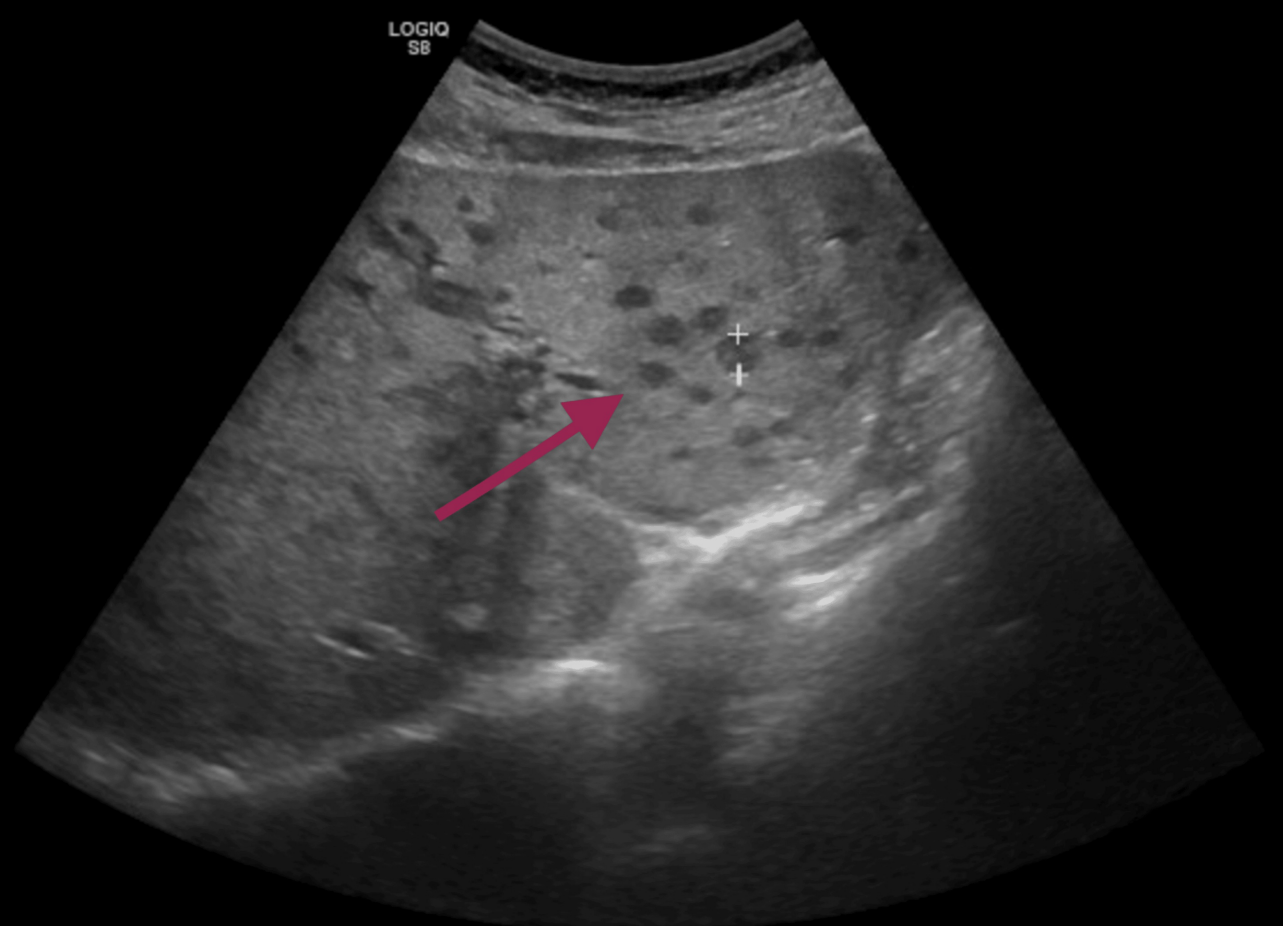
Abdominal ultrasound showing multiple hepatic nodules suggestive of infeccious foci Abdominal ultrasound showing hepatic nodules that were first thought to be infection but later found to be metastases, after biopsy.
